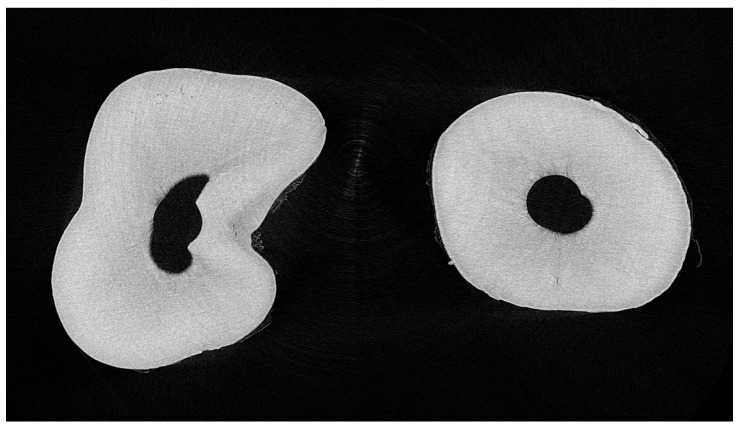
The middle layer of the cervical third in the EDTA group shows changes in root canal morphology made via the ultrasonic instrument.
